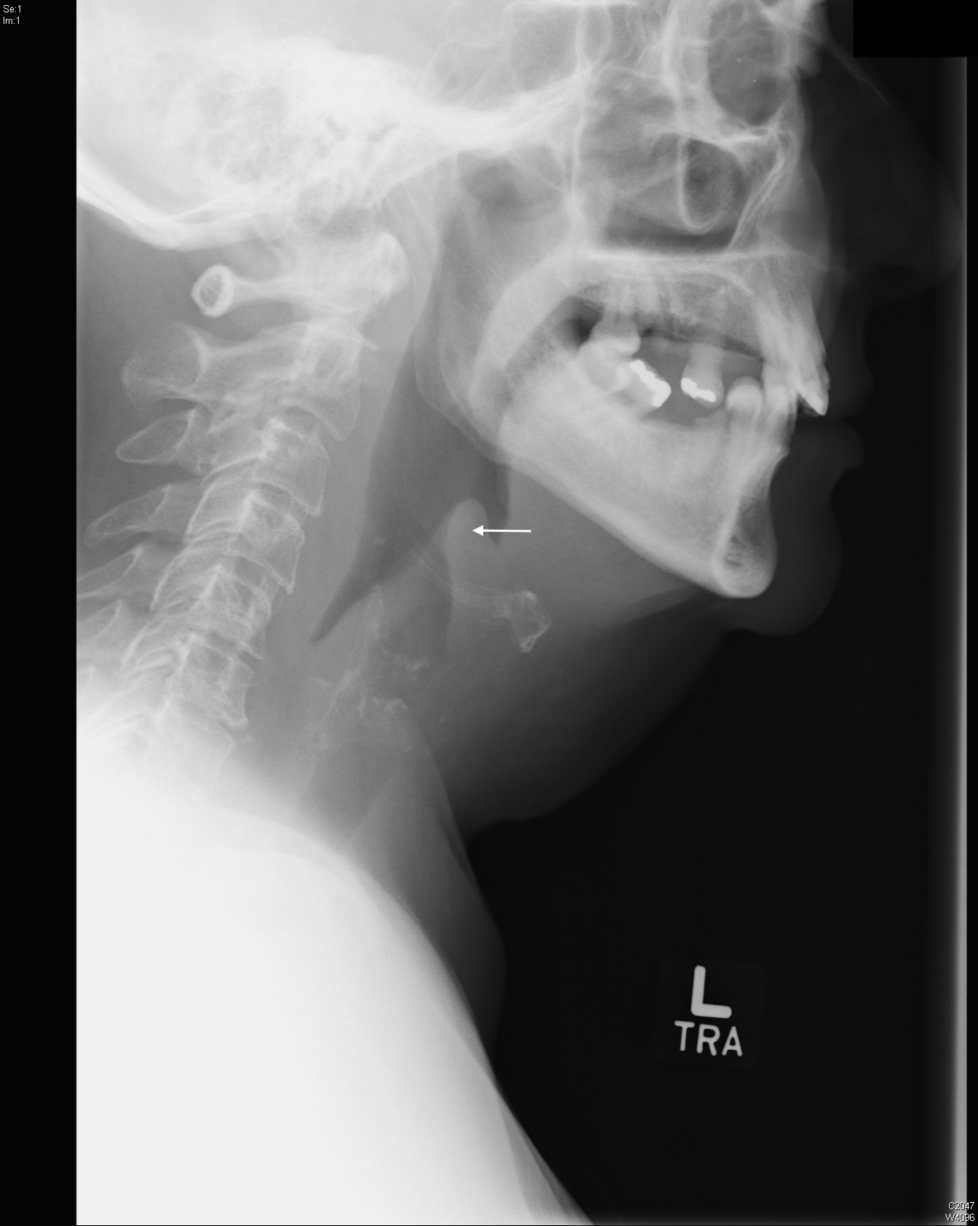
Radiograph demonstrating enlarged epiglottis and aryepiglottic folds.
